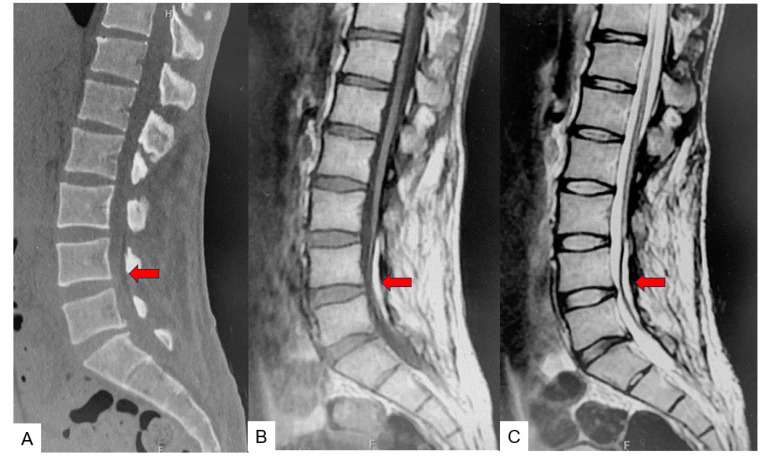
Preoperative CT and MR imaging. (A) Mid sagittal 3D reconstruction CT. (B) T1 weighted mid-sagittal MR imaging. (C) T2 weighted mid-sagittal MR imaging. A lipoma is attached to the spinal cord and retethering is observed (red arrows).
